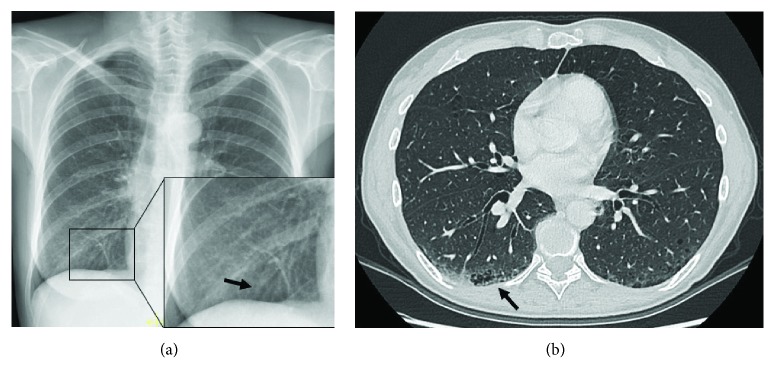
A 55-year-old woman with CD. Chest X-ray shows right basal bronchial wall thickening suggesting bronchitis (a). Axial HRCT study shows peribronchial wall thickening associated with bronchiolectasis (arrow) and signs of subpleural emphysema (b).
